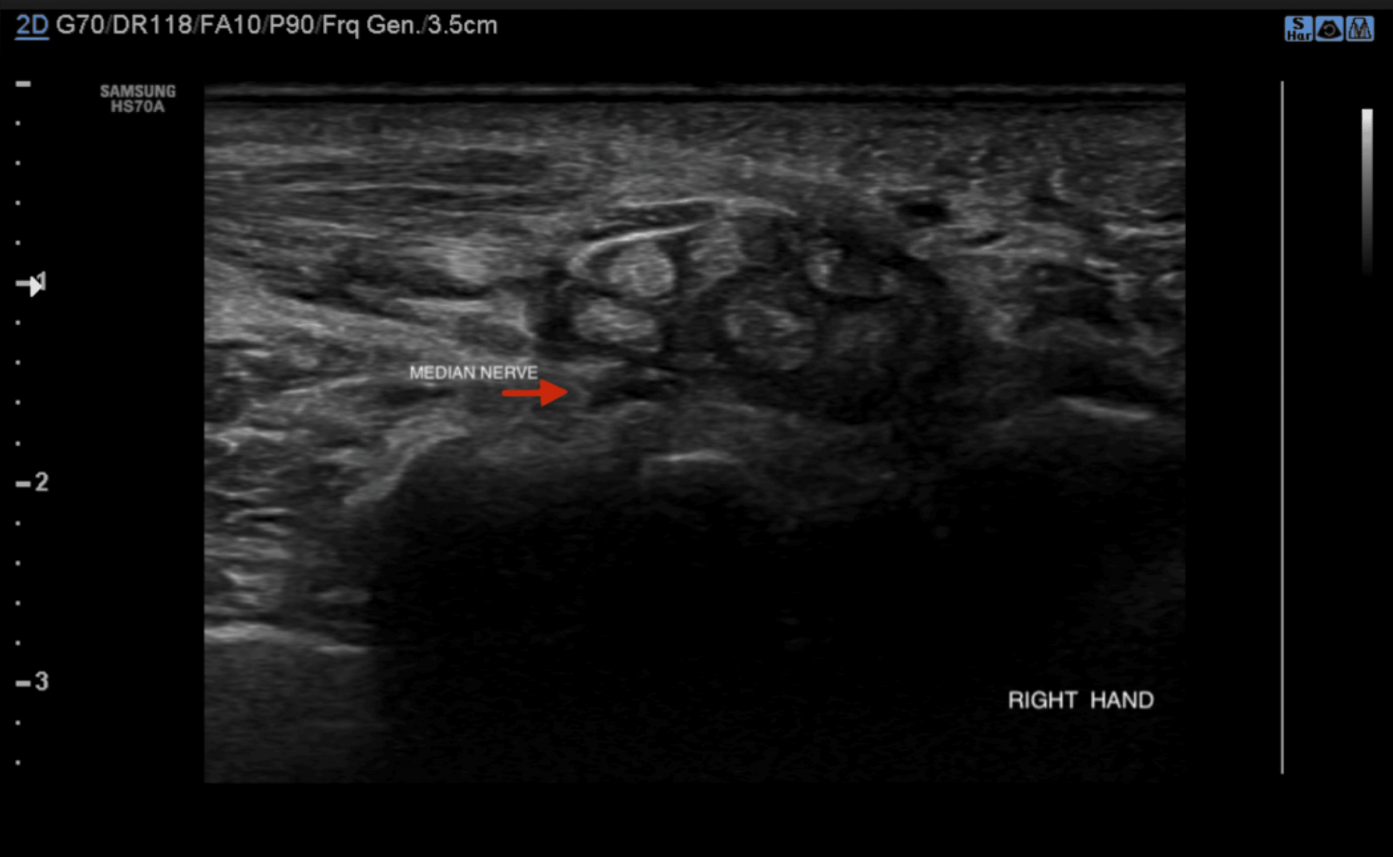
High-resolution ultrasonography of the right median nerve at the wrist High-resolution ultrasonography of the right median nerve showing median nerve in relation to flexor retinaculum. Loss of fascicular architecture and echotexture of the nerve with flattening of the nerve can be observed with palmar bowing of the flexor retinaculum. Flexor and extensor tendons appear bulky and echogenic with surrounding fluid, indicating the likelihood of tendinitis.
